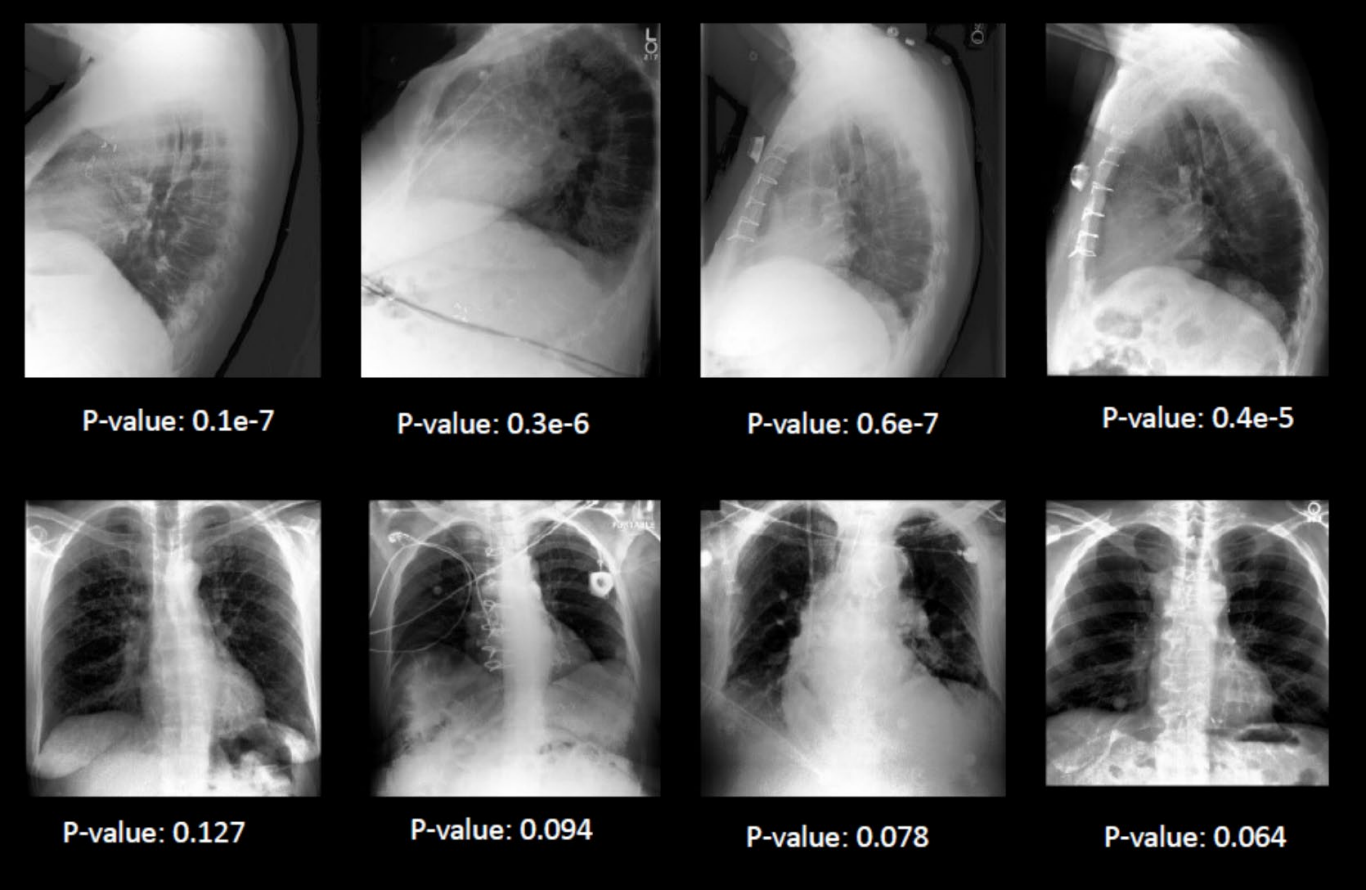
Several examples of frontal and lateral chest radiographs with successful discrimination performance (as denoted by the *p*-values)
